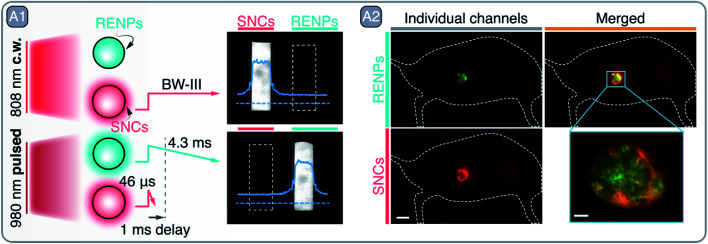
A – Multiplexed imaging with RENPs and SNCs: A1 – graphical depiction of RENPs and SNCs PL behavior under continuous wave (c.w.) 808 nm and pulsed 980 nm excitation. NIR images of RENPs and SNCs dispersions under c.w. and pulsed excitation are also shown, sole detection of RENPs PL under pulsed 980 nm excitation is achieved by implementing 1 ms delay, which filters out short-lived PL of SNCs. A2 – two-plexed imaging of CT-26 tumor with RENPs and SNCs. Adapted by permission from Springer Nature: Springer Nature Nature Biotechnology ref. 96, © 2019.
